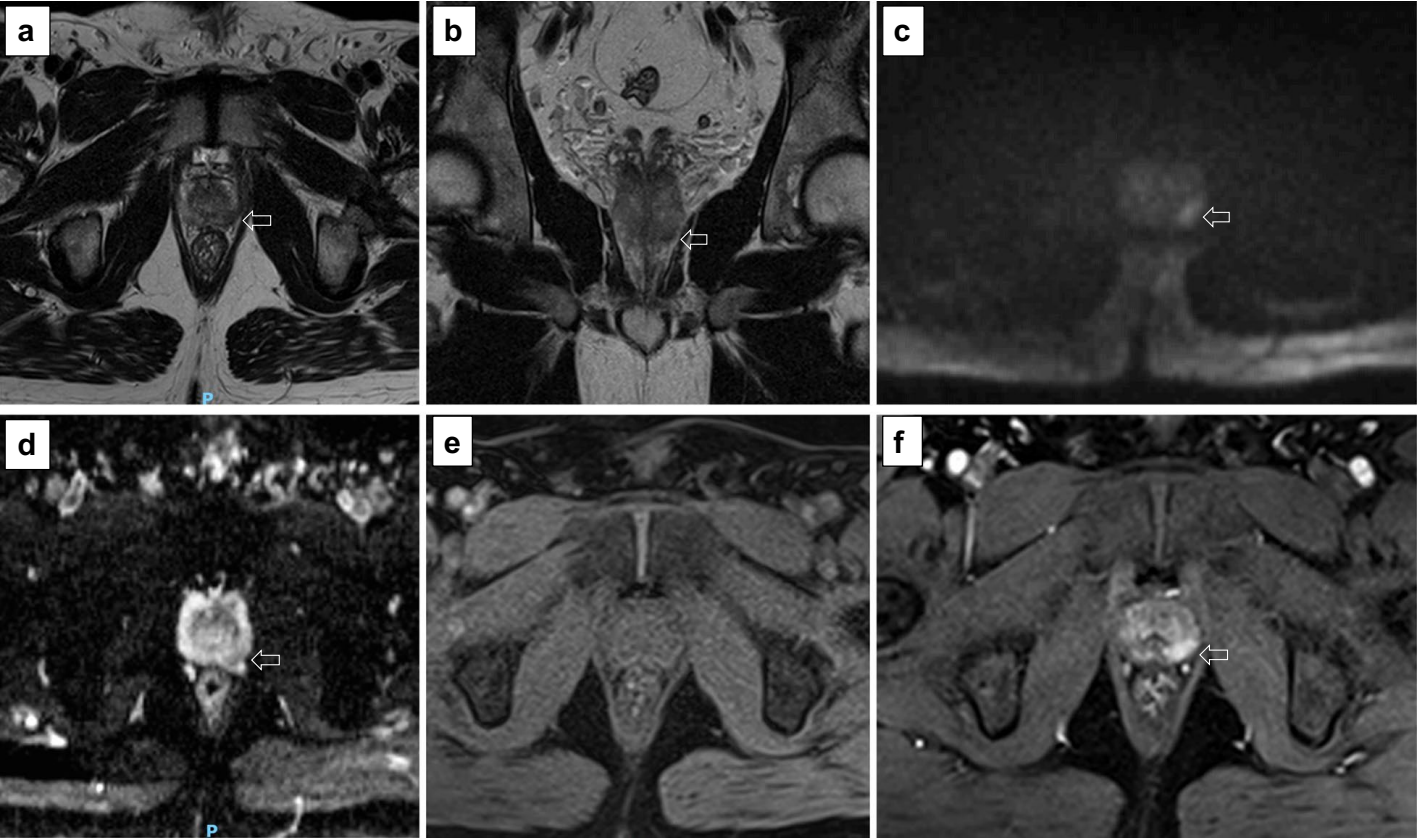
Axial (a) and coronal (b) T2w imaging, high *b* value (c), ADC map (d) and pre-contrast (e) and DCE acquisitions (f) of a study that was given a PI-QUAL score of 3. At least two MR sequences taken together are of diagnostic quality: although T2w imaging is of good diagnostic quality, some parameters are not compliant with the PI-RADS v.2.1 technical recommendations such as the field of view for DWI (23 × 31 cm in c, d—please note that the DWI images here are magnified) and the temporal resolution (17 s) of DCE sequences. The arrows indicate a lesion (Likert 4/5) in the left peripheral zone between 4 and 5 o’clock. Targeted biopsy revealed Gleason 3 + 4 disease
